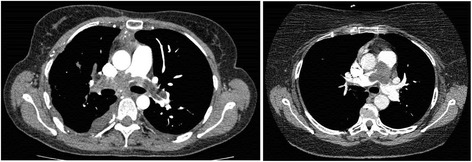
Pulmonary artery intimal sarcoma mimicking pulmonary embolism. CT images of two patients who presented with dyspnoea and dependent oedema.
